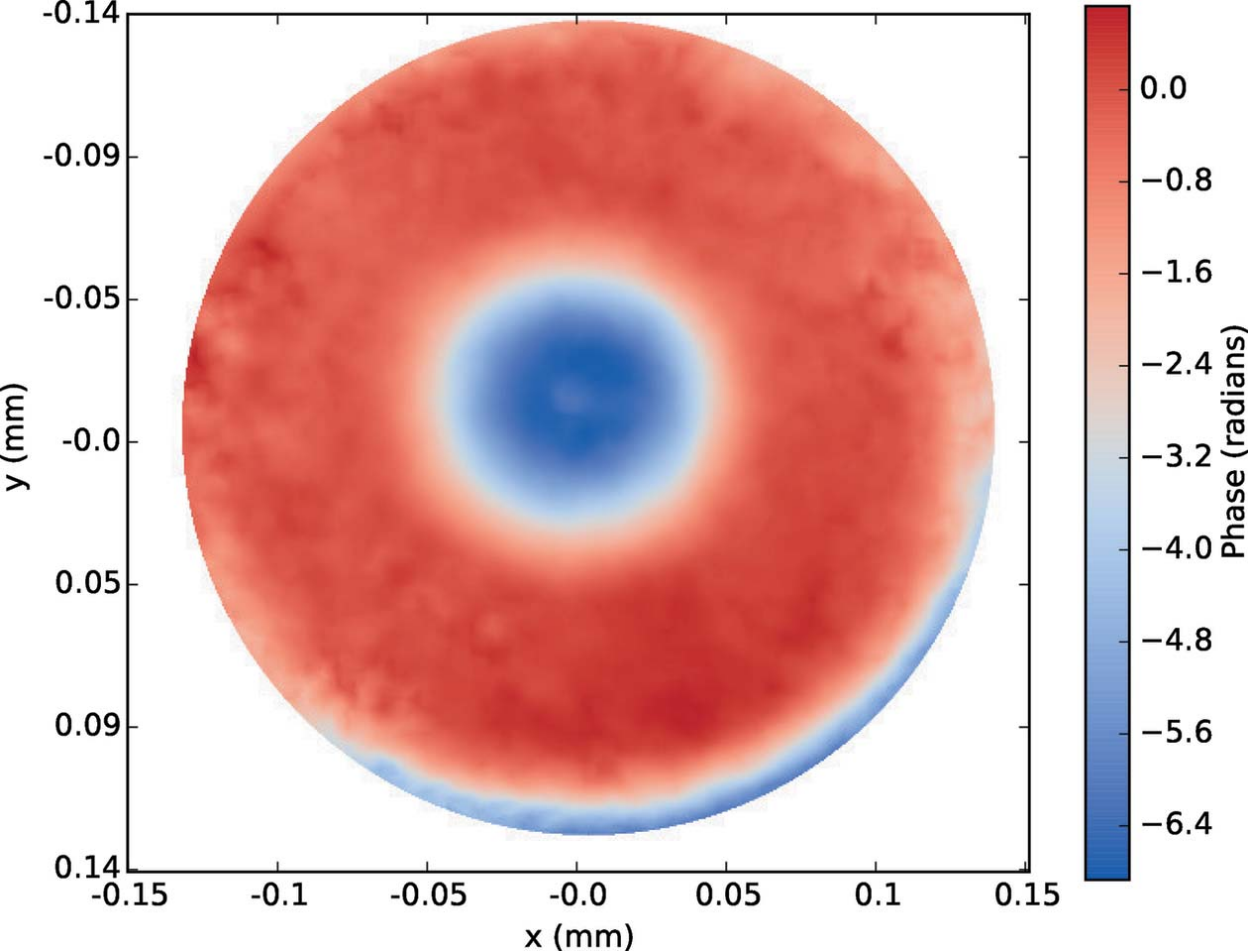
Recovered profile of the full phase plate, based on X-ray speckle tracking in differential mode. This shows the full contribution of the phase plate to the wavefront, spherical term included. Note that the plate was not well centered at this time.
